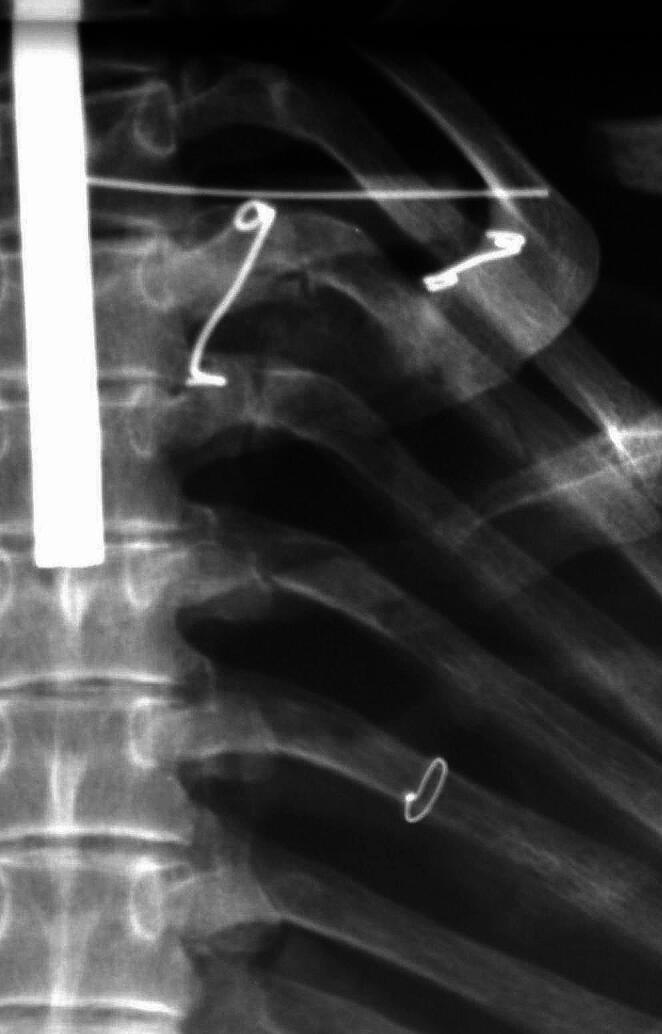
Chest x-ray of the right upper thorax. This shows the ring-like wire fixation of rib 6 identical to other post-mortem fixations of the skeleton (rib, apical scapula, vertebra)
